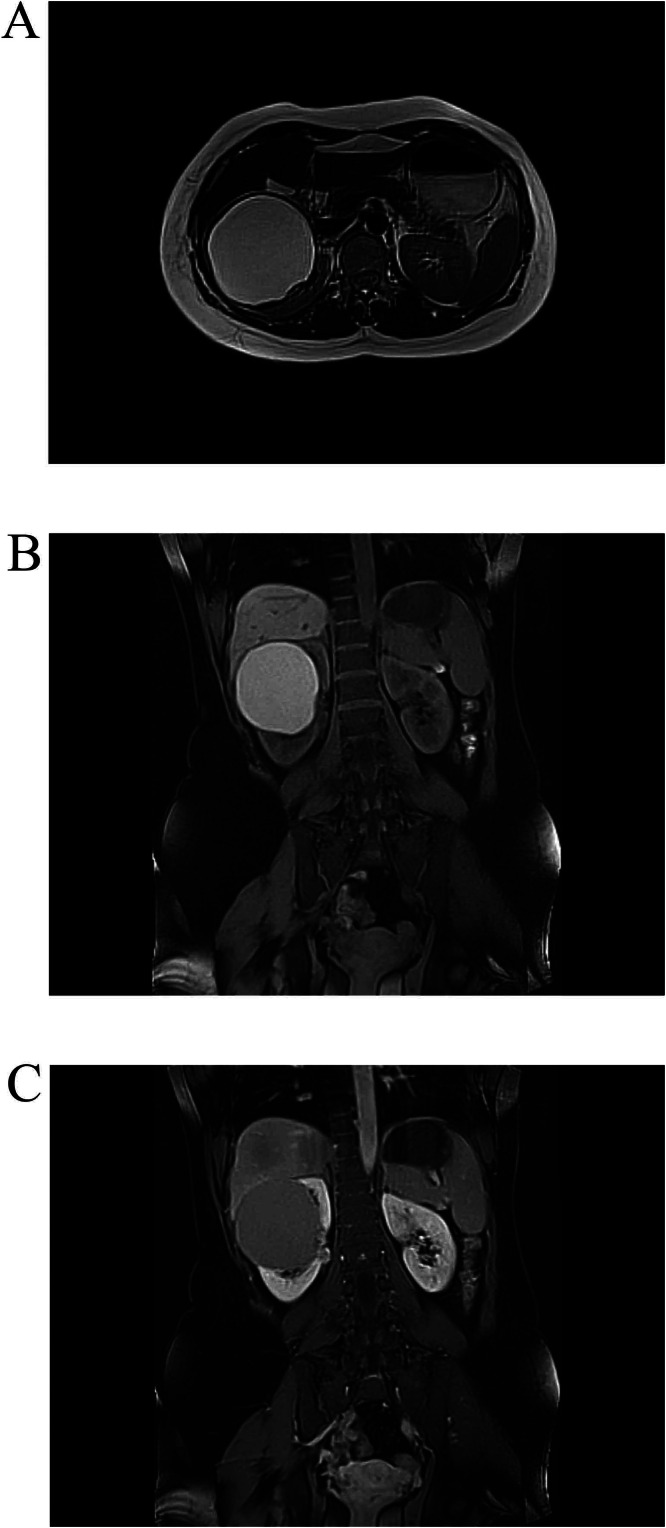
The right renal cystic lesion appeared hyperintense on axial T1WI. A) hyperintense signal on axial T2 WI. B) No signal loss on precontrast coronal fat suppression T1WI, and no restriction on DWI (not shown). C) No obvious enhancement in postcontrast coronal fat suppression T1WI.
